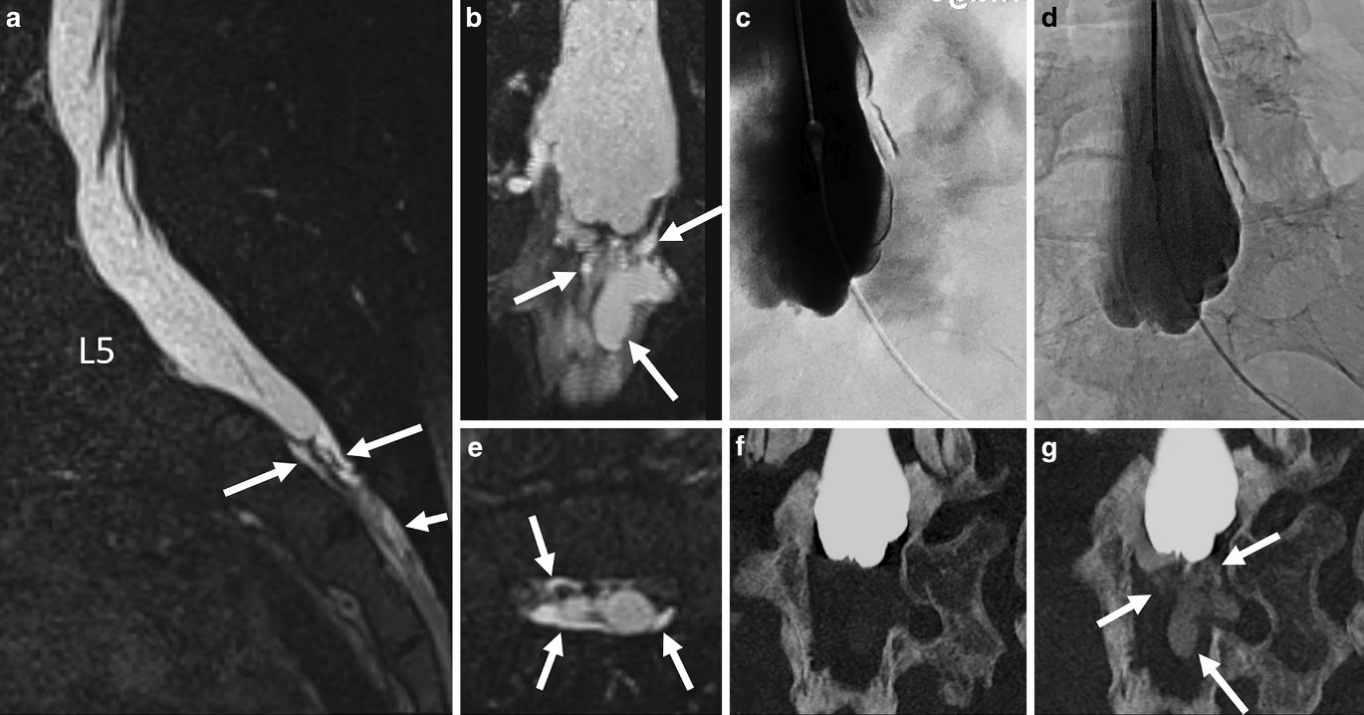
40-year-old woman with a subtle sacral leak and SIH-score of 6. Sagittal, coronal and axial 3D T2-MRM show a small SLEC at the sacral level (*arrows* in a, b, e). Linear fluid indicates the extradural component of SLEC (*arrows* in e). Neither DSM in left lateral decubitus and reversed Trendelenburg positions(c) nor delayed single X‑ray in anterior-posterior projection reveal contrast outflow (d). Coronal CT myelography in supine position does not show epidural contrast outflow with certainty (f) but delayed CT scan 30 min after walking around does (*arrows* in g), representing the sacral origin of leak
